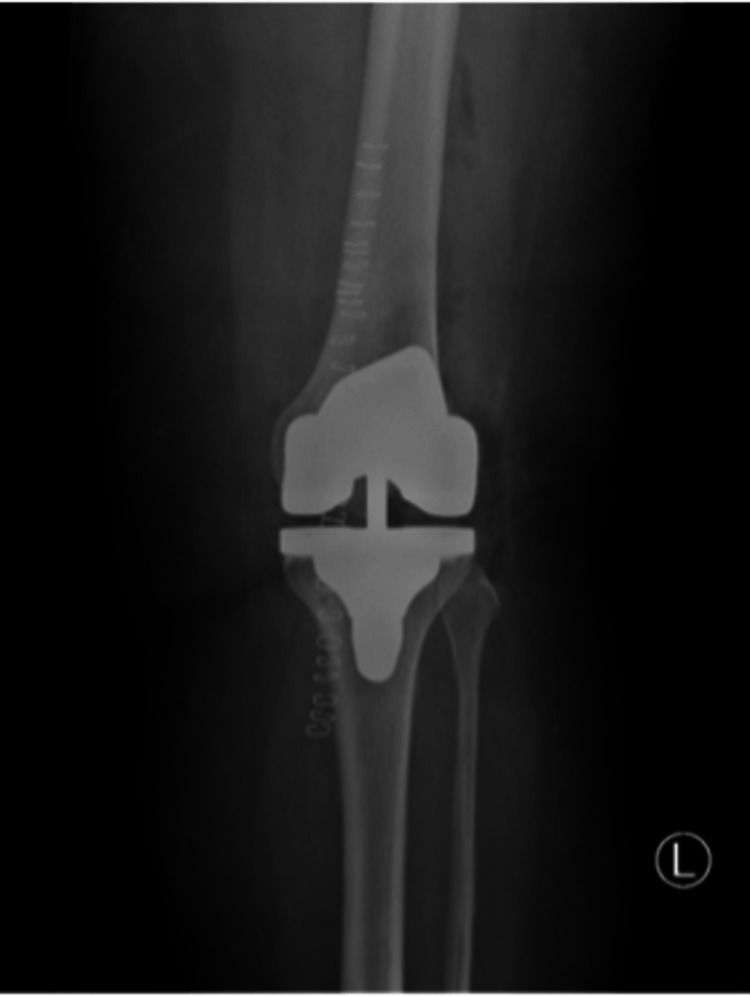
Left knee X-ray after total knee arthroplasty, with no postoperative complications
